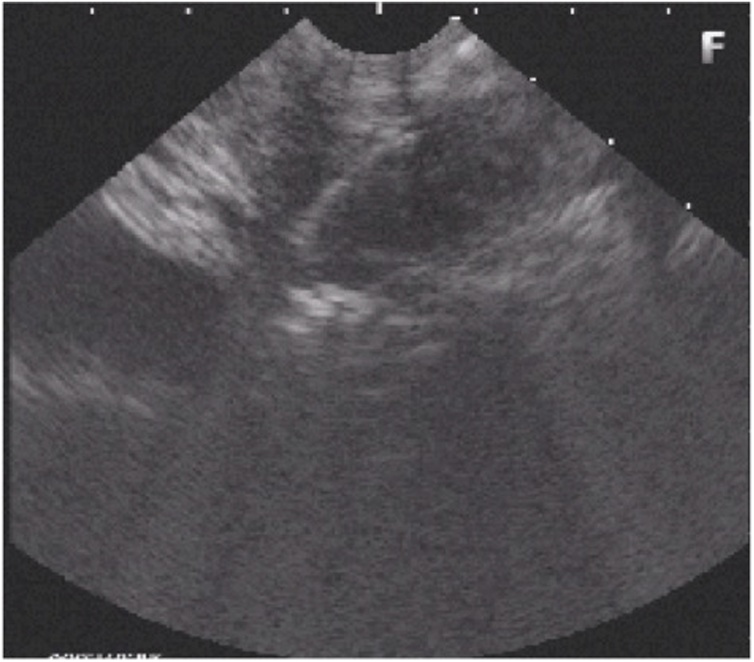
Endoscopic ultrasound. Endoscopic ultrasound showed a 31.5 × 9.1 mm subepithelial anechoic oval lesion in distal lateral esophageal wall, 37 cm from incisors.
